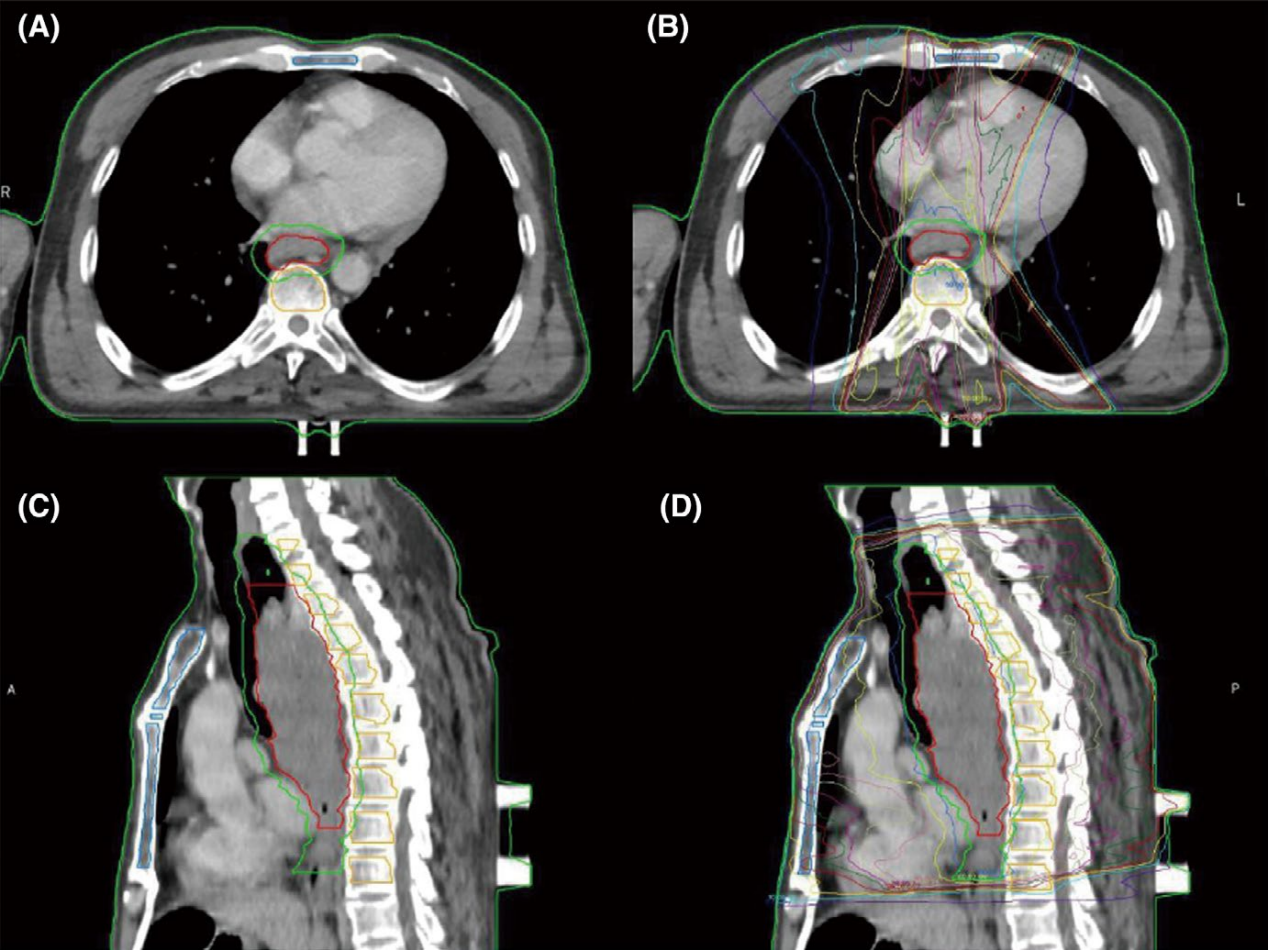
Delineation on cross‐sectional (A, B) and sagittal planes (C, D) CT. (B) and (D) show the isodose line of target. Structures included tumor PTV (green), GTV (red), vertebra body BM (yellow), and sternum BM (blue)
